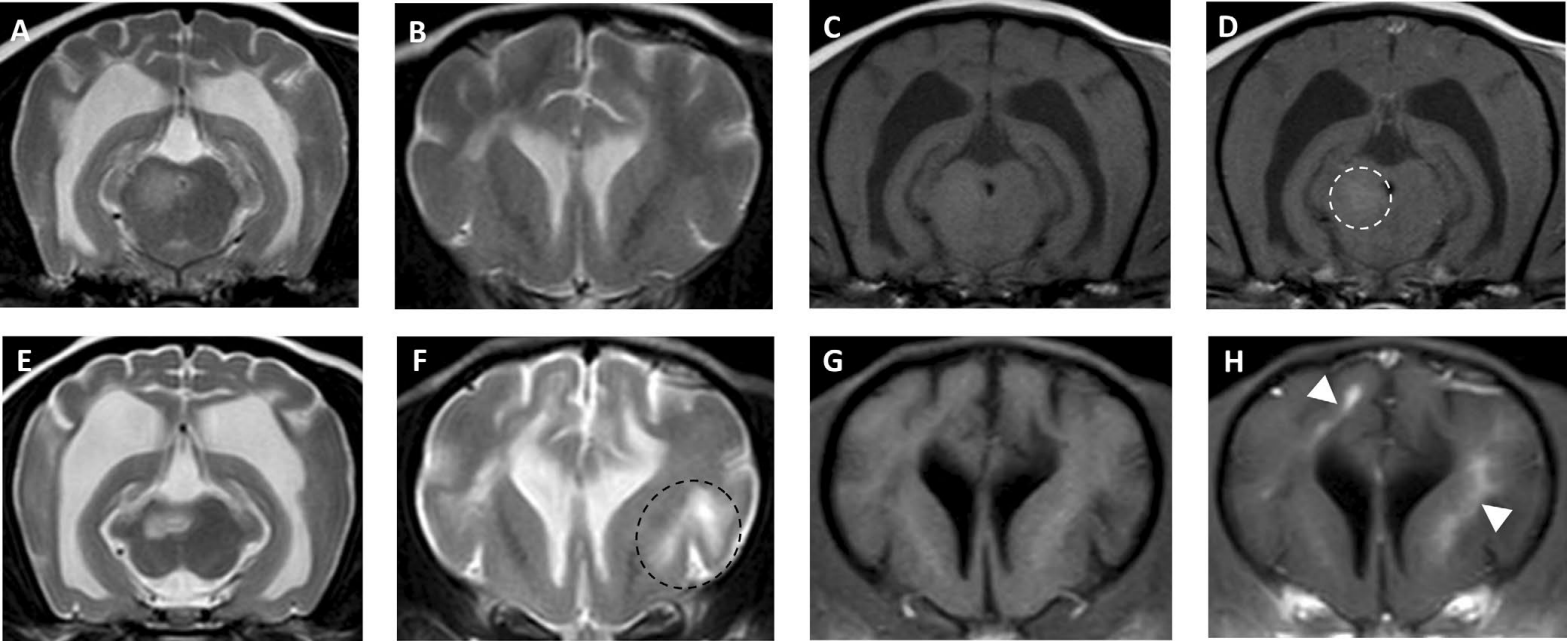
Magnetic resonance imaging of a 3‐year‐old crossbreed with MUO that showed clinical improvement but remained with permanent neurological deficits at the time of initial diagnosis of MUO (A–D) and 12‐months later (E–F). Images (A–B) and (E–F) are transverse T2‐weighted (T2W) images, showing enlargement of the lateral ventricles and sulci, suggesting parenchymal atrophy and also development of a new lesion in the frontal cortex (black dotted circle) not seen in the initial MRI. Images (C) and (G) are transverse T1‐weighted (T1W) images and (D) and (H) are transverse T1W post‐contrast images showing the only mildly contrast enhancing lesion in the initial MRI (dotted white circle) and the new areas of contrast enhancement in the second MRI (white arrowheads).
